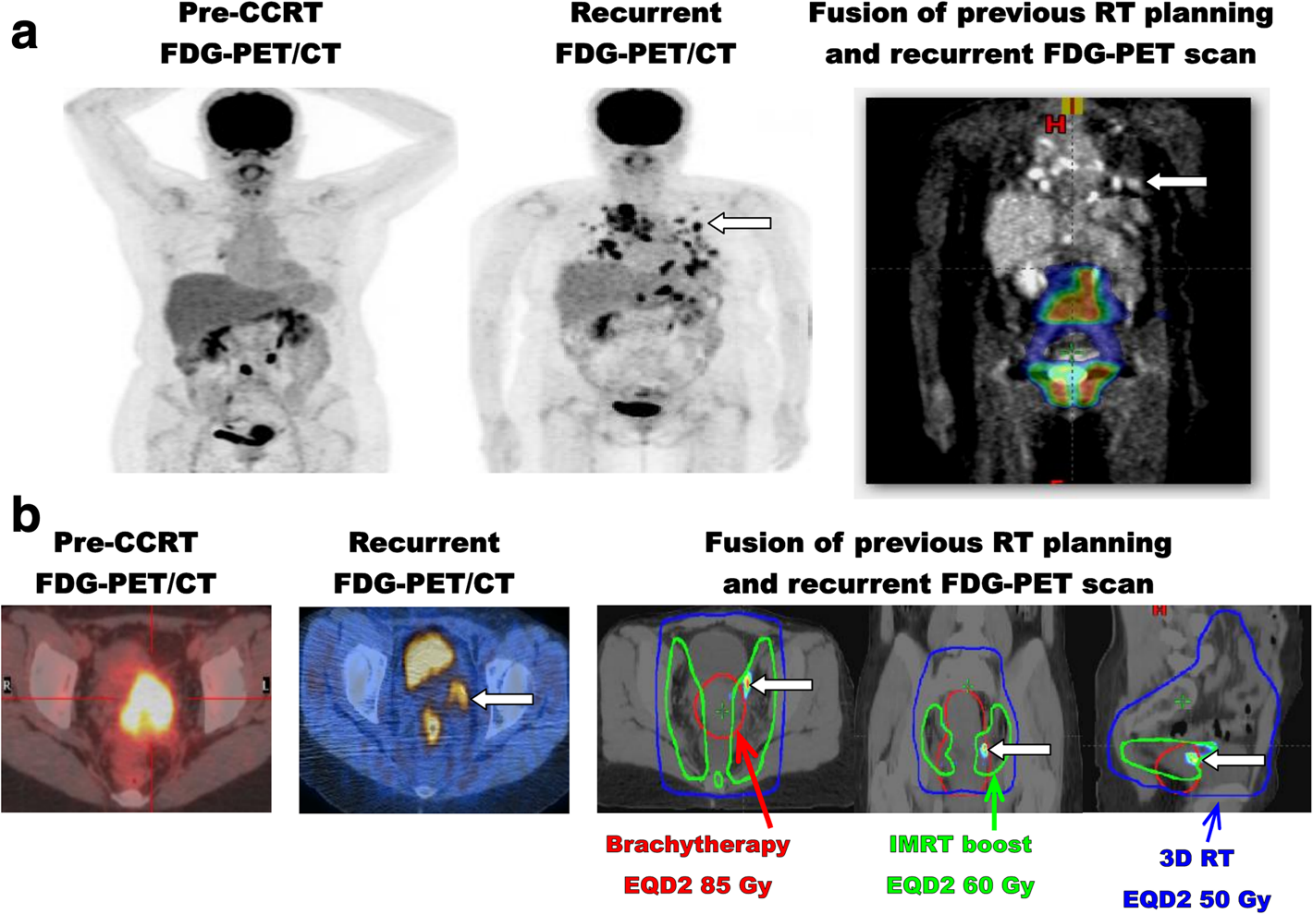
Patterns of failure after ^18^F-FDG PET-guided RT planning. Pre-treatment combined RT planning scans of 3D-RT, IMRT and 3D-brachytherapy are fused to post-treatment recurrent ^18^F-FDG PET/CT scans to map the recurrent tumors in the initial RT treatment fields and dose distribution. The doses of external beam radiation and brachytherapy are transformed to EQD2 (equivalent dose to a 2-Gy fraction) for combination. a Out-of field recurrence and distant metastasis. RT dose distribution is demonstrated by colors. The lung metastasis confirmed by pathology is indicated by a white arrow. Note that the post-RT in-field structures show lower metabolic activity as compared to those in the pre-RT scan. (b) In-field recurrence. Note that the FDG-avid recurrent cervical tumor (white arrow) confirmed by pathology is located at the junctional zone of IMRT (EQD2 60 Gy) and brachytherapy (EQD2 85 Gy) in the parametrium
